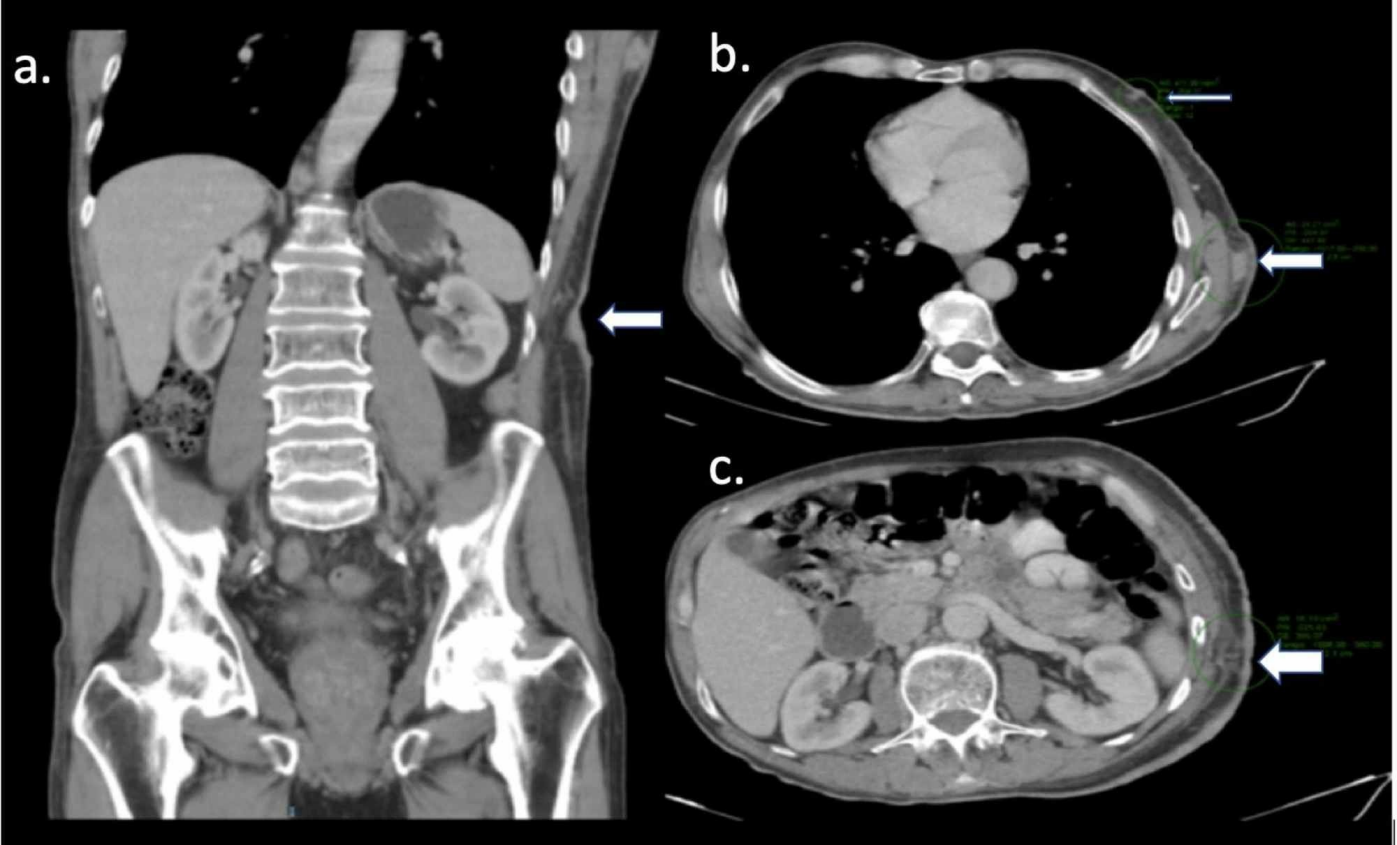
CT scan of the abdomen showing thickening of the skin and metastatic subdermal nodules (normal arrows on panels a - b) and ulcerated skin and soft tissue edema (thick arrow on panel c)
